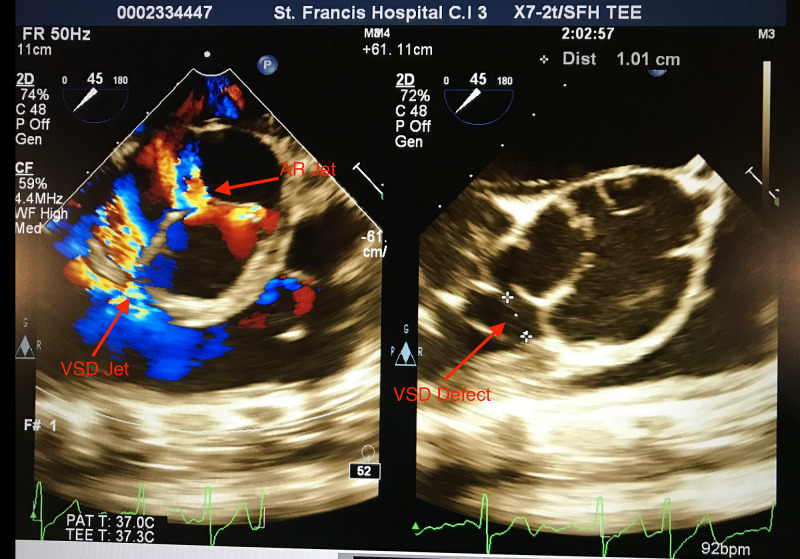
TEE showing the location of VSD jet and AR jet TEE- transesophageal echocardiogram; VSD- ventricular septal defect; AR- aortic regurgitation
